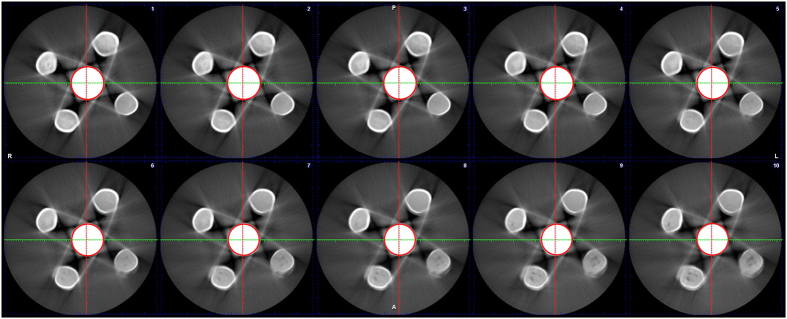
The sphere boundary on ten two-dimensional CBCT images of one dental material with clear artefacts.
